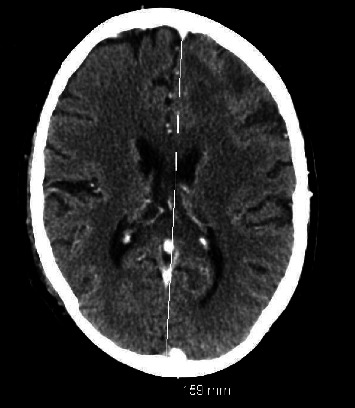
Follow-up axial CT head without contrast after 3 days showed left frontal horn mass effect and 3–4 mm of left-to-right shift of the third ventricle. White line showing midline shift.
